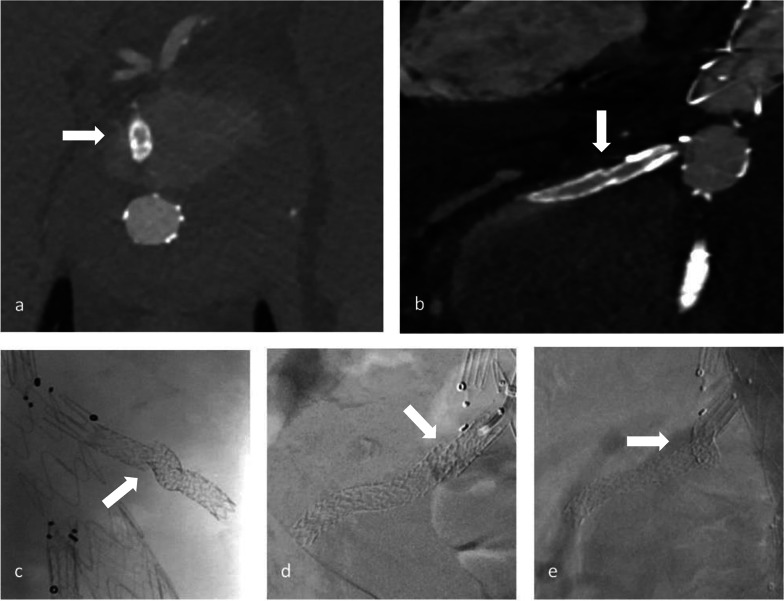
An unspecific endoleak in CTA (a) was found to be a complete separation of the SMA BSG in angiography (c–e). Only after retrospectively calculated CT reformatations (b), the BSG fracture is visible. During catheterization, the dislocation of the distal fragment precluded endovascular repair
